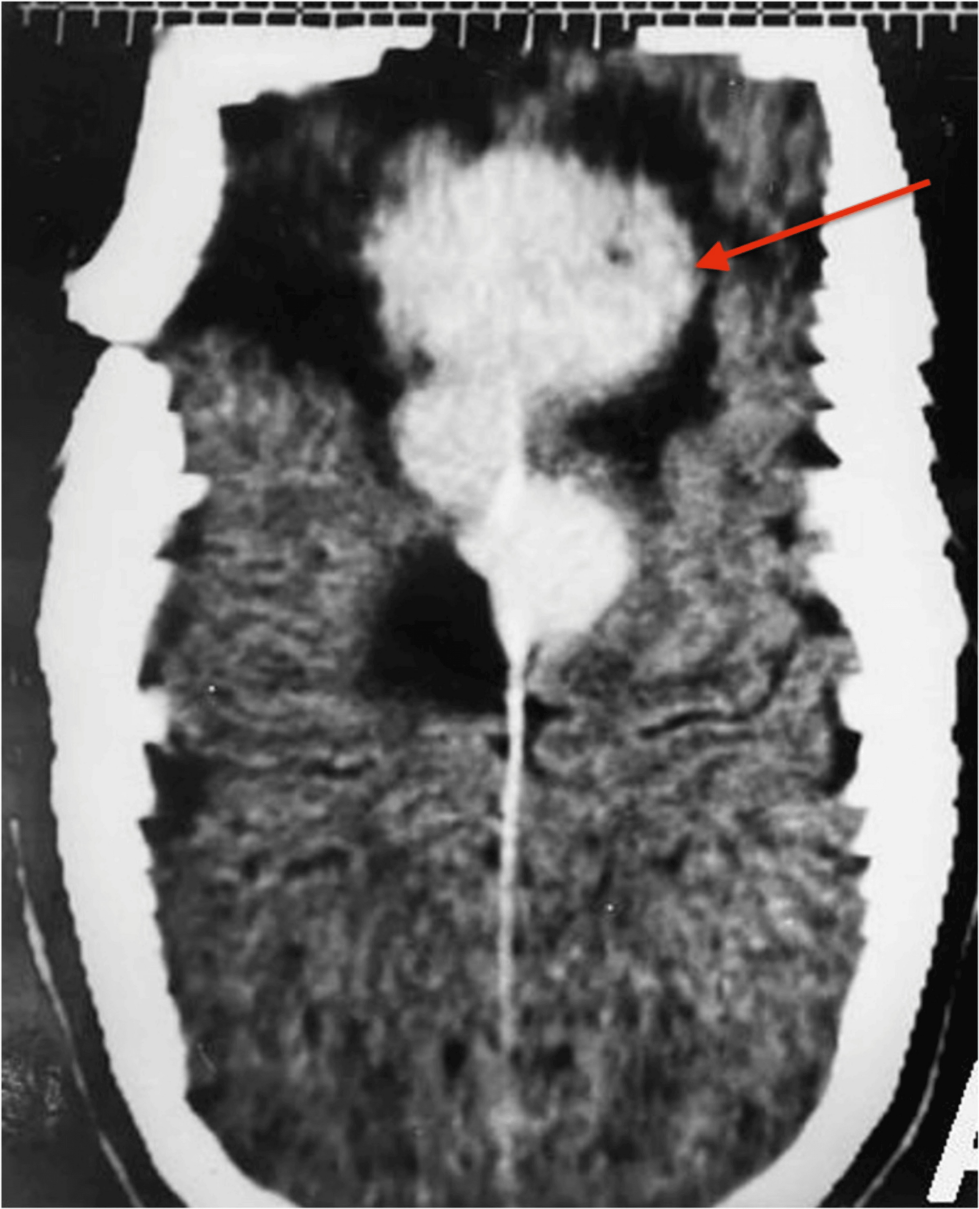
In the puerperium, the brain CT scan showed a recurrence of the tumor (red arrow)
